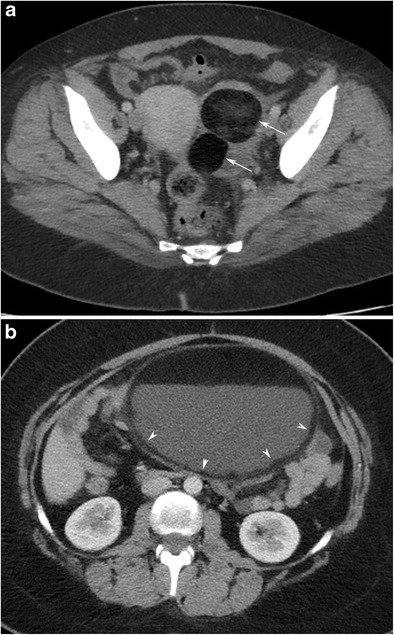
Axial CT image of a 25-year-old female with mature cystic teratoma (MCT). Two round fatty structures (arrows) are seen in the left adnexal mass (a). In another case with the fat-fluid level in an MCT, the axial CT image (b) shows a thin fat layer covering the inner side of the wall (arrowheads)
